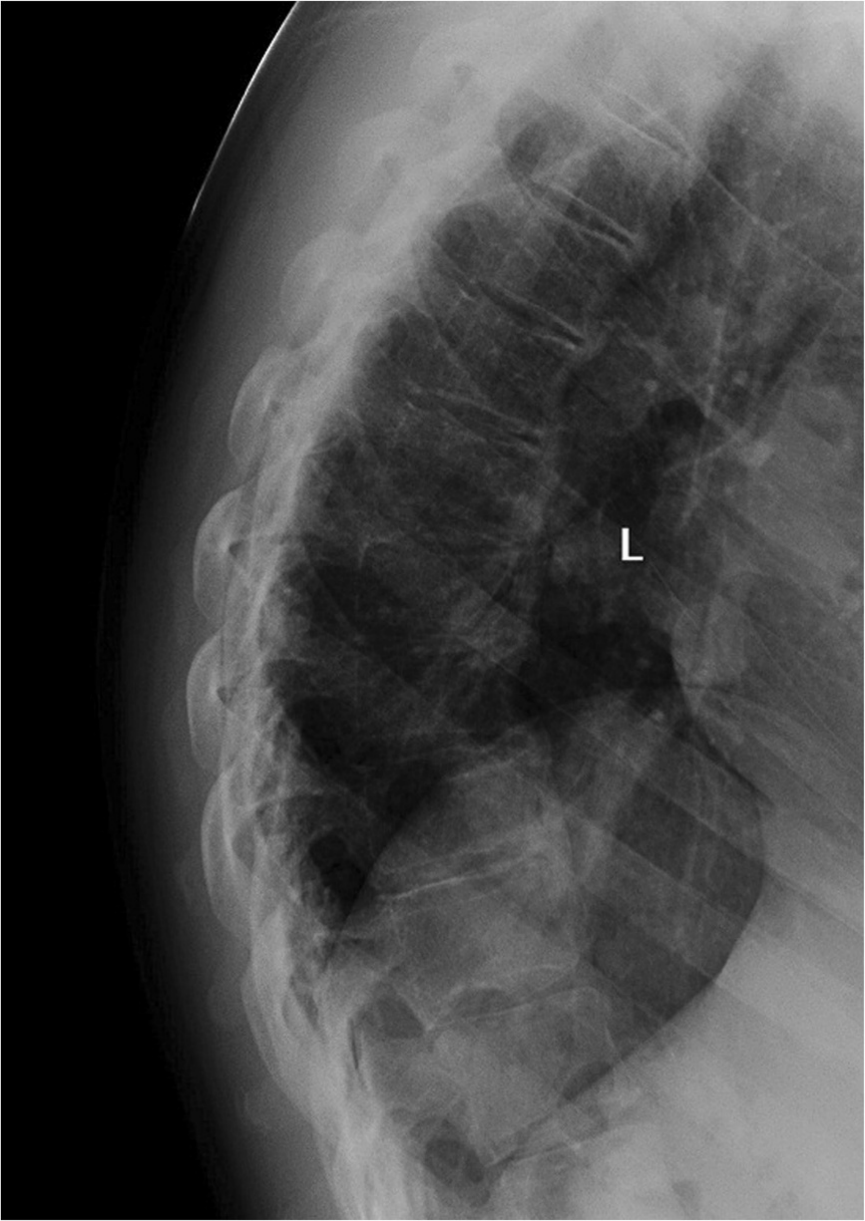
Lateral X-RAY showing flowing ossification along mid-inferior aspects of the thoracic spine.
